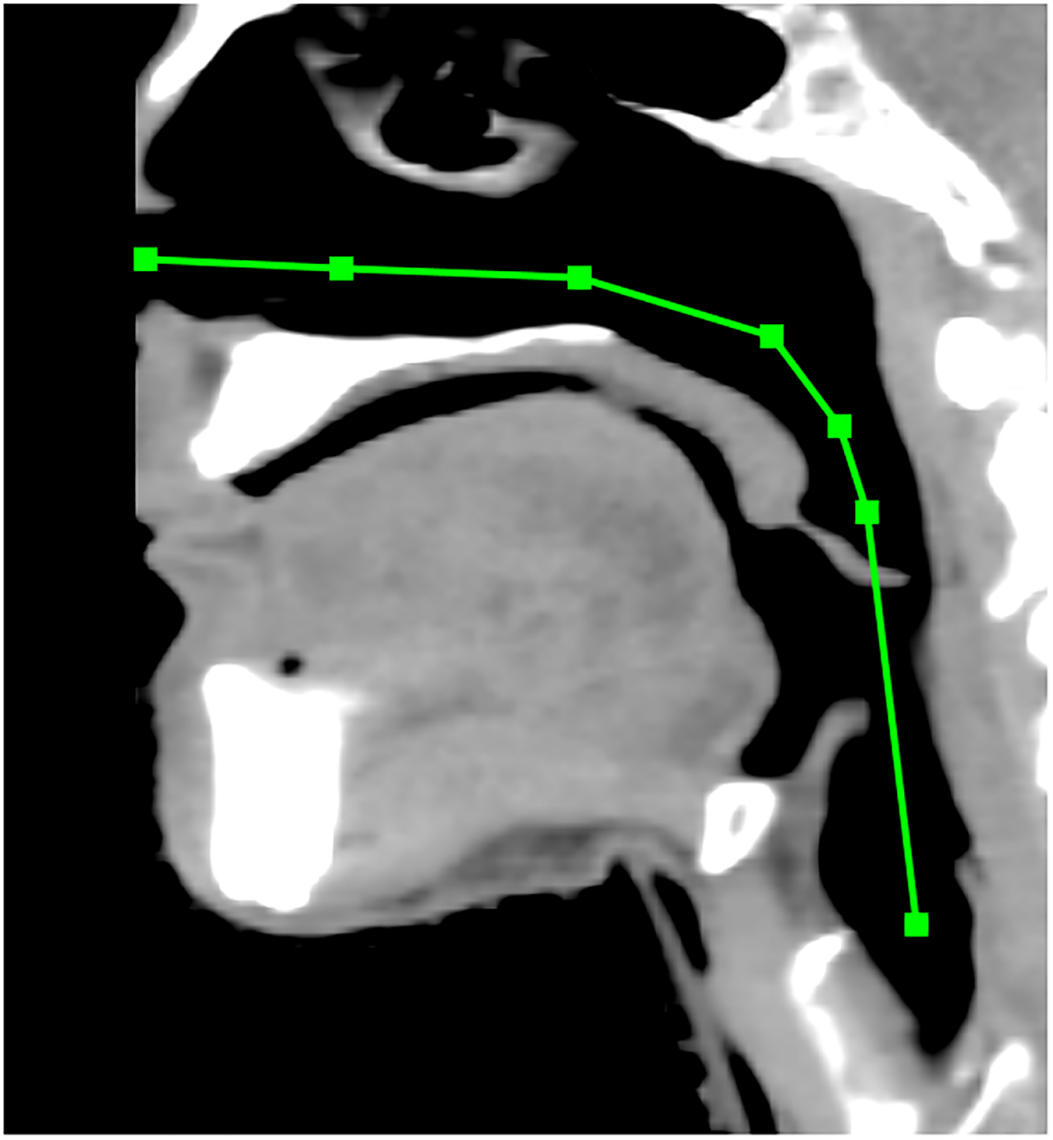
Virtual endoscope path creation in a patient. A sagittal CT slice shows the manually-selected virtual endoscope path through a patient used for the Location Search registration technique. The nose is cropped out due to the field-of-view reduction described in section 2.4.3.
